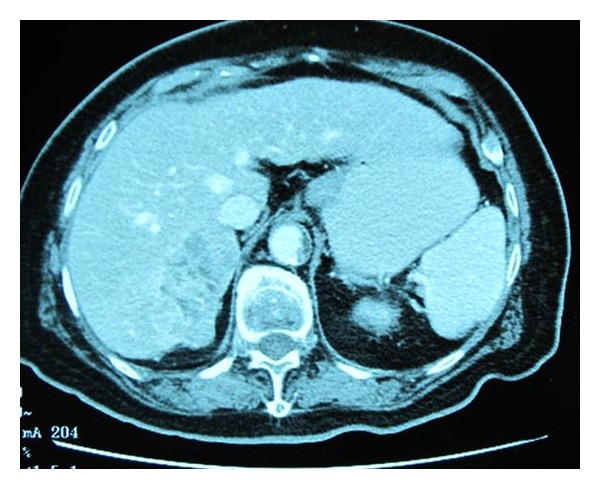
CT scan showing heterogeneous vascular right adrenal mass, suggestive of a pheochromocytoma.
